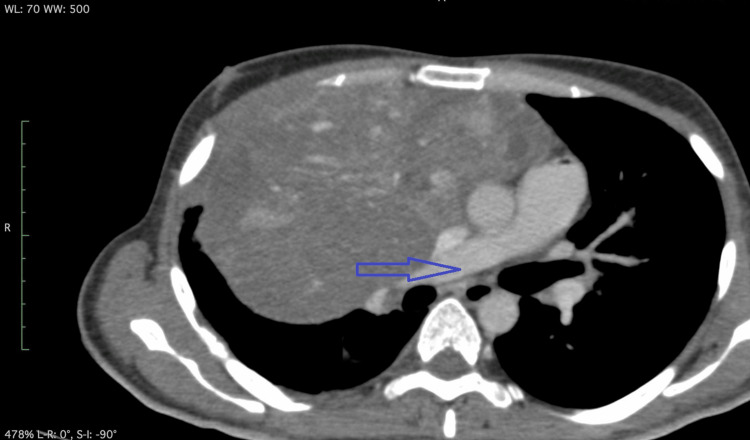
CTPA showing no evidence of thromboembolism. Axial image from CTPA demonstrating the main pulmonary artery (blue arrow) with no filling defects. indicating the absence of pulmonary embolism. CTPA: computed tomography pulmonary angiogram
